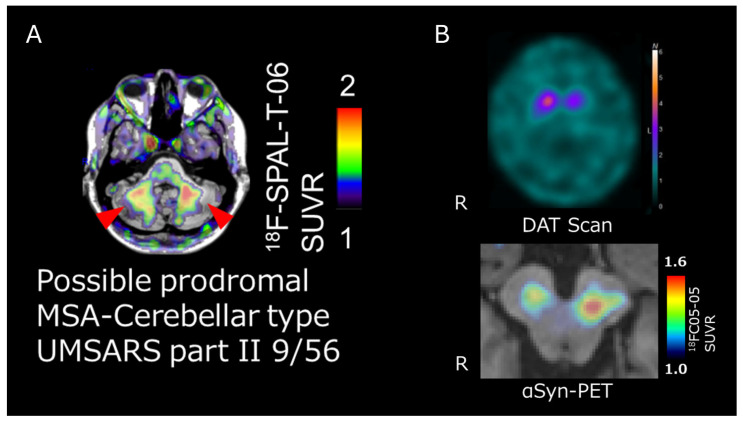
α-Synuclein PET imaging in vivo. (A). Axial SUVR PET image of [^18^F]SPAL-T-06 overlaid on MRI in a patient with possible prodromal multiple system atrophy of cerebellar type (MSA-C). Shown at the level of the middle cerebellar peduncles, the tracer accumulates in regions characteristic of MSA (the pons, middle cerebellar peduncles, and cerebellar white matter), achieving high-contrast visualization of α-synuclein pathology (red arrowheads). (B) Concordance between striatal DAT-SPECT uptake reduction (upper panel) and [^18^F]-C05-05 accumulation in the midbrain (lower panel), both showing left-predominant changes contralateral to the right-dominant motor symptoms, in a patient with familial PD due to the A53T mutation in the SNCA gene.
